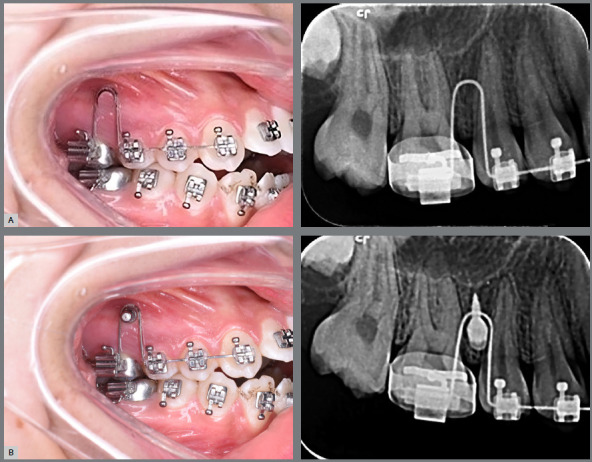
Intraoral photographs and periapical radiographs with the location guide before insertion of the miniscrew (A) and after insertion of the miniscrew (B).
